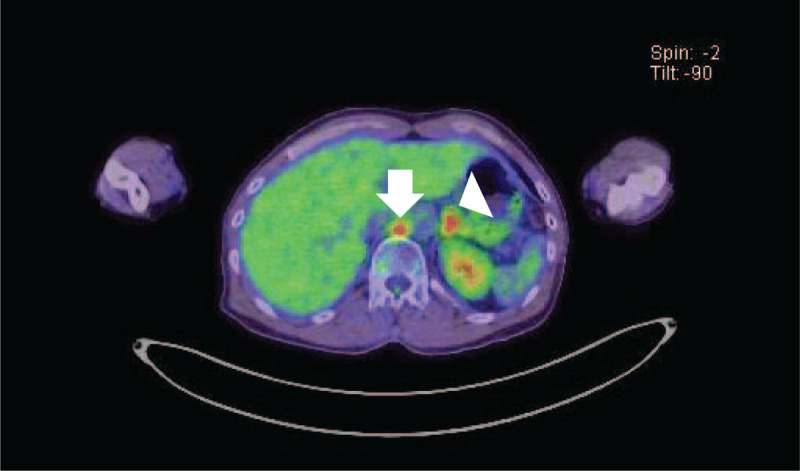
Positron emission tomography and computed tomography showing enlarged adrenal gland (arrowhead) and para-aorta lymph node (arrows).
